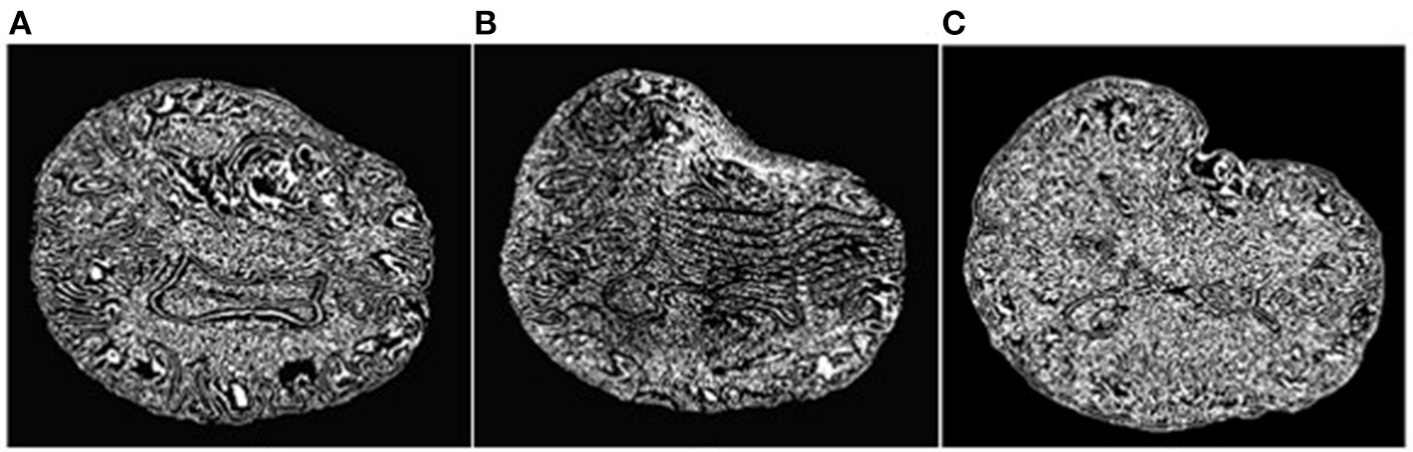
Extracted Canny points in a single slice for quantitative evaluation of registration accuracy using the HD metric. (A): Points extracted from the preoperative MRI; (B): Points of (A) after transformation to the intraoperative space; (C): Points extracted from the intraoperative MRI. The HD metric is computed between point sets (B) and (C). Note that the Canny points are generally different from feature points used for registration.
